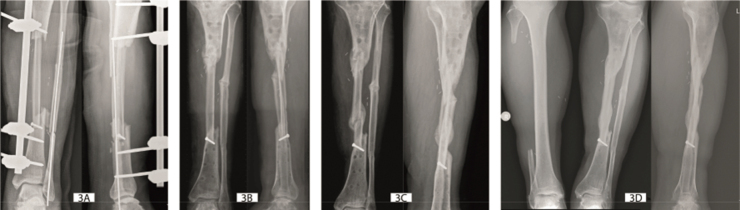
A. Tibial diaphyseal reconstruction following a crush injury due to a farm accident. B. X-rays taken during the 5^th^ postoperative month reveal a solid union. C. X-rays taken during the 8^th^ postoperative month reveal a healing stress fracture of the flap. D. X-rays taken during the 25^th^ postoperative month show hypertrophy of the entire flap, which is more pronounced at the fracture site.
